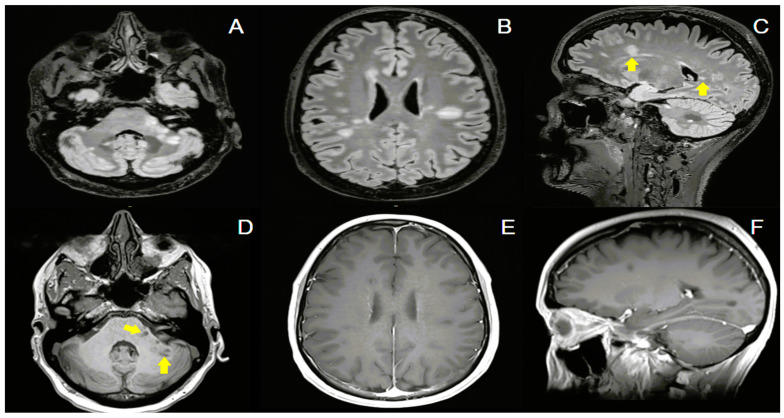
Brain MRI following radiation therapy. FLAIR images showed several hyperintense ovoid lesions in both infratentorial (A) and supratentorial regions (periventricular and subcortical/juxtacortical) (B,C). Several of these lesions are oriented perpendicular to the long axis of the lateral ventricles ((C), arrows). A few hypointense lesions on T1-MPRAGE images, consistent with “black holes”, are depicted in image (D) (arrows). On T1-weighted post-contrast imaging (E,F), there is no evidence of gadolinium enhancement. Abbreviations: FLAIR: fluid-attenuated inversion recover; MPRAGE: magnetization prepared rapid gradient echo.
